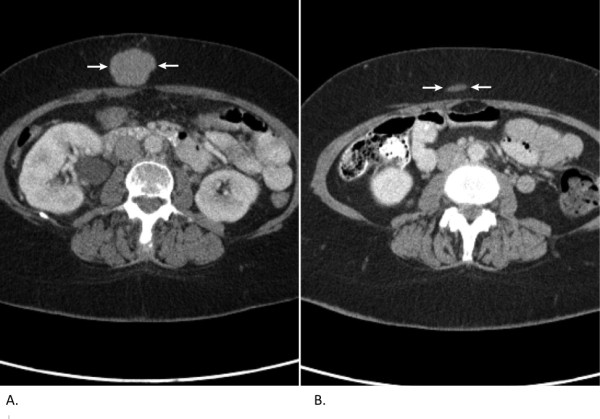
Anterior abdominal subcutaneous tumor. Subcutaneous abdominal mass (source of diagnostic biopsy). (A) Subcutaneous mass at initial diagnosis (white arrows). (B) Marked reduction in subcutaneous mass following three cycles of chemotherapy (white arrows). The CT scan slices are aligned to show the maximum dimensions of the soft tissue tumor mass.
